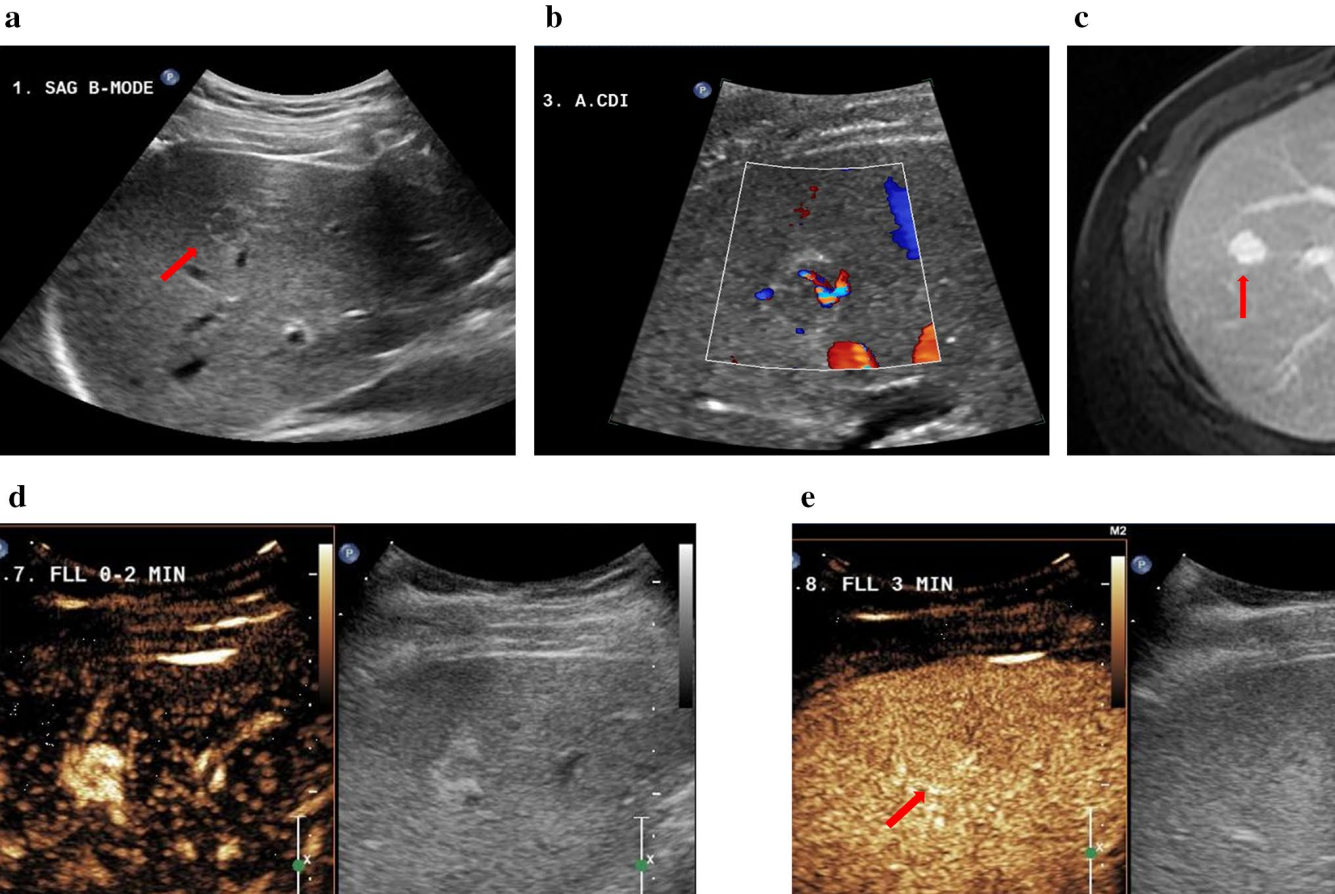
34-year-old male with focal nodular hyperplasia (FNH). a Pre-contrast harmonic B-mode showing the lesion. b In Colour Doppler imaging the lesion shows central vascularity and some peripheral vascularity. c The hyperintense representation of the lesion in the venous/delayed phase on CE-MRI. Imaging performed post-SonoVue injection. d Arterial phase shows well-defined lesion margins and central scarring. e FNH homogeneous iso-enhancement indicated in the late phase at 3 min
